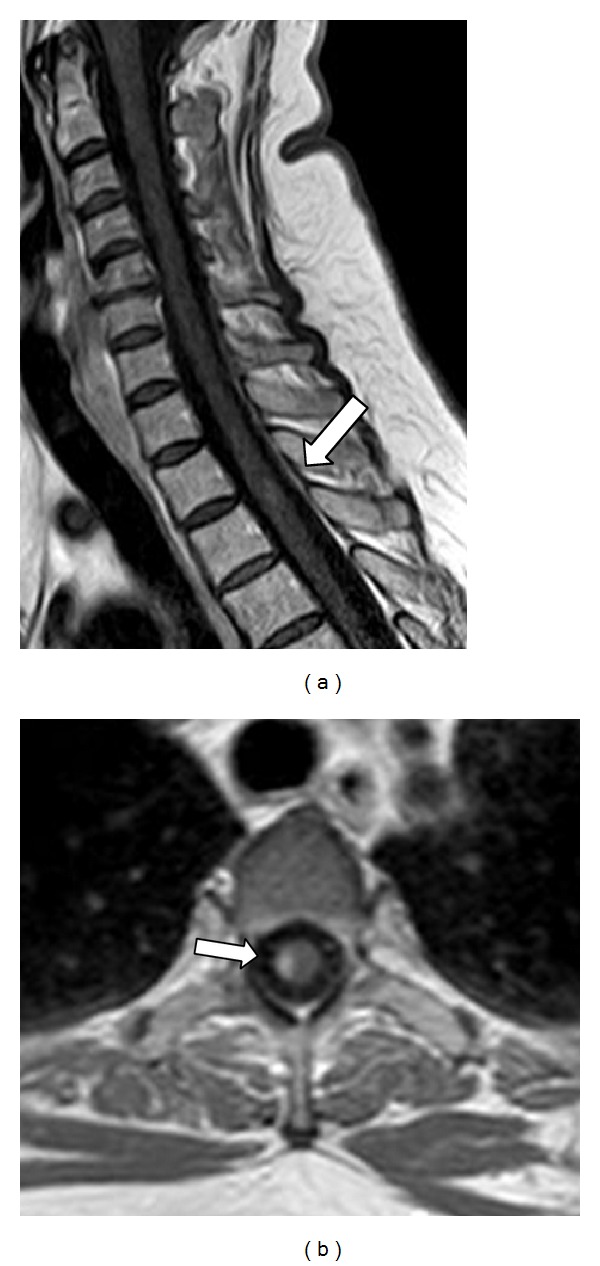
(a) Sagittal and (b) axial T1-weighted cervical/thoracic MRI showing contrast enhanced lesion at the T2-T3 level.
